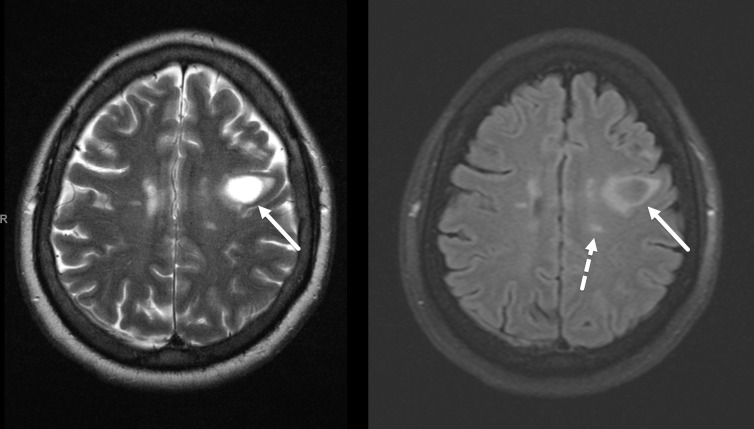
Apparent T2-FLAIR mismatch sign in a 46-year-old male with no significant past medical history. Axial T2 (left) and FLAIR (right) MR images demonstrate a 3 cm T2 hyperintense lesion in the left posterior frontal lobe (solid arrows). There is FLAIR signal suppression of the lesion, similar in appearance to the T2-FLAIR mismatch sign. Additionally, there are oval T2 hyperintense foci in the periventricular white matter in roughly perpendicular orientation to the ventricles (dashed arrow). FLAIR, fluid attenuated inversion recovery.
